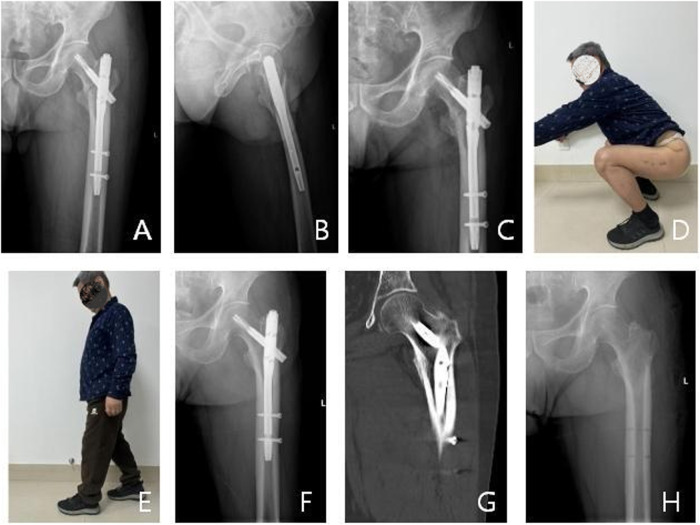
Postoperative follow-up imaging. (A) Anteroposterior radiograph of the left femur on postoperative day 2. (B) Lateral radiograph of the left femur on postoperative day 2. (C) Anteroposterior radiograph of the left hip at 6 weeks postoperatively. (D) Clinical photograph showing hip flexion at 6 months postoperatively. (E) Clinical photograph demonstrating standing and walking posture at 6 months postoperatively. (F) Radiograph obtained at 1 year postoperatively showing healed fracture site. (G) Coronal computed tomography (CT) image of the left femur at 1 year postoperatively. (H) Radiograph obtained 1 year postoperatively after removal of the internal fixation device. (Patient's facial features are pixelated to protect confidentiality.)
